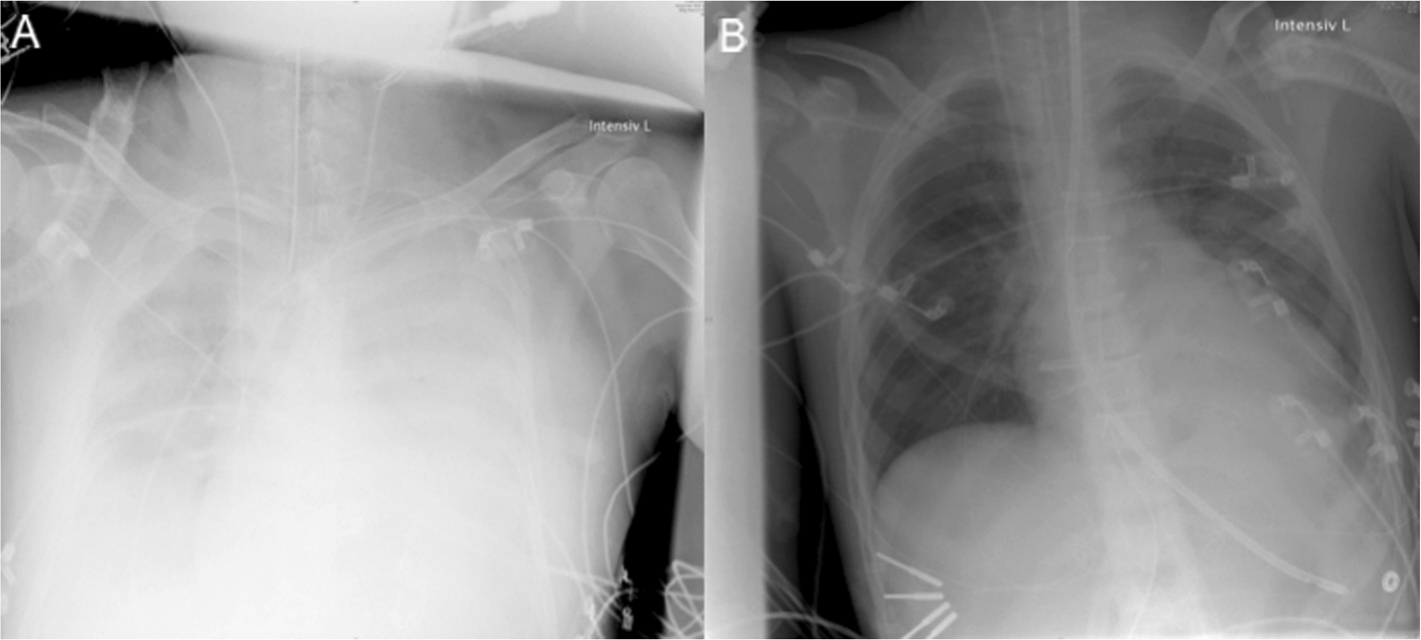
Anteroposterior chest x-ray of a typical patient of our study cohort with cardiogenic shock before (A) and after (B) initiation of ECLS with left ventricular decompression. The system rapidly induces a significant decrease in pleural effusion and pulmonary congestion.
